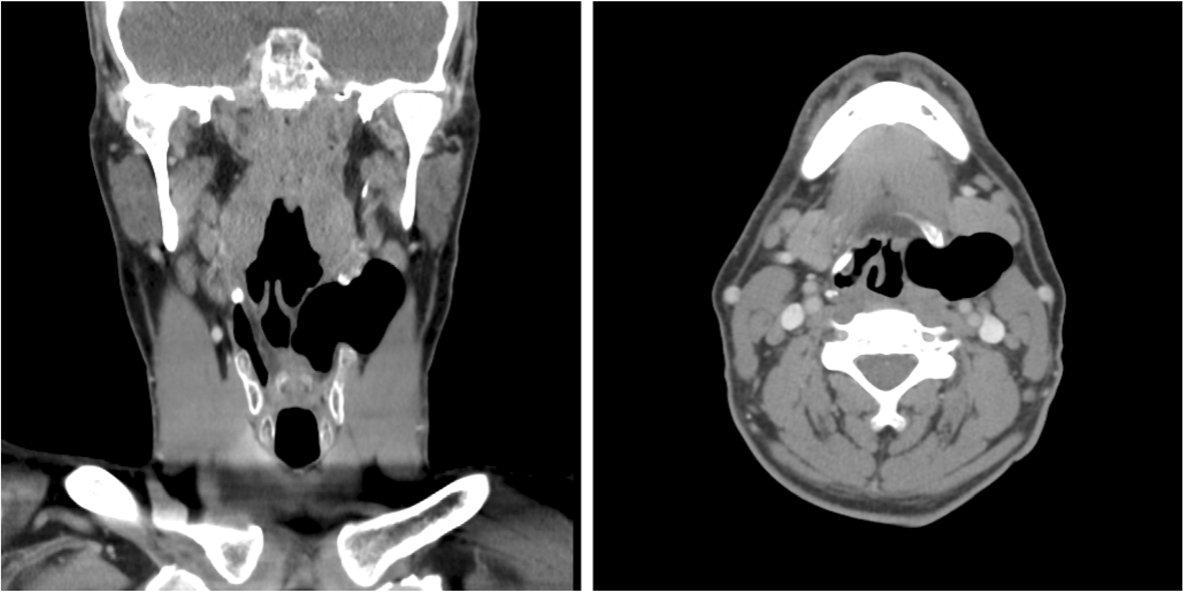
CT of the neck with contrast demonstrating a laryngocele
